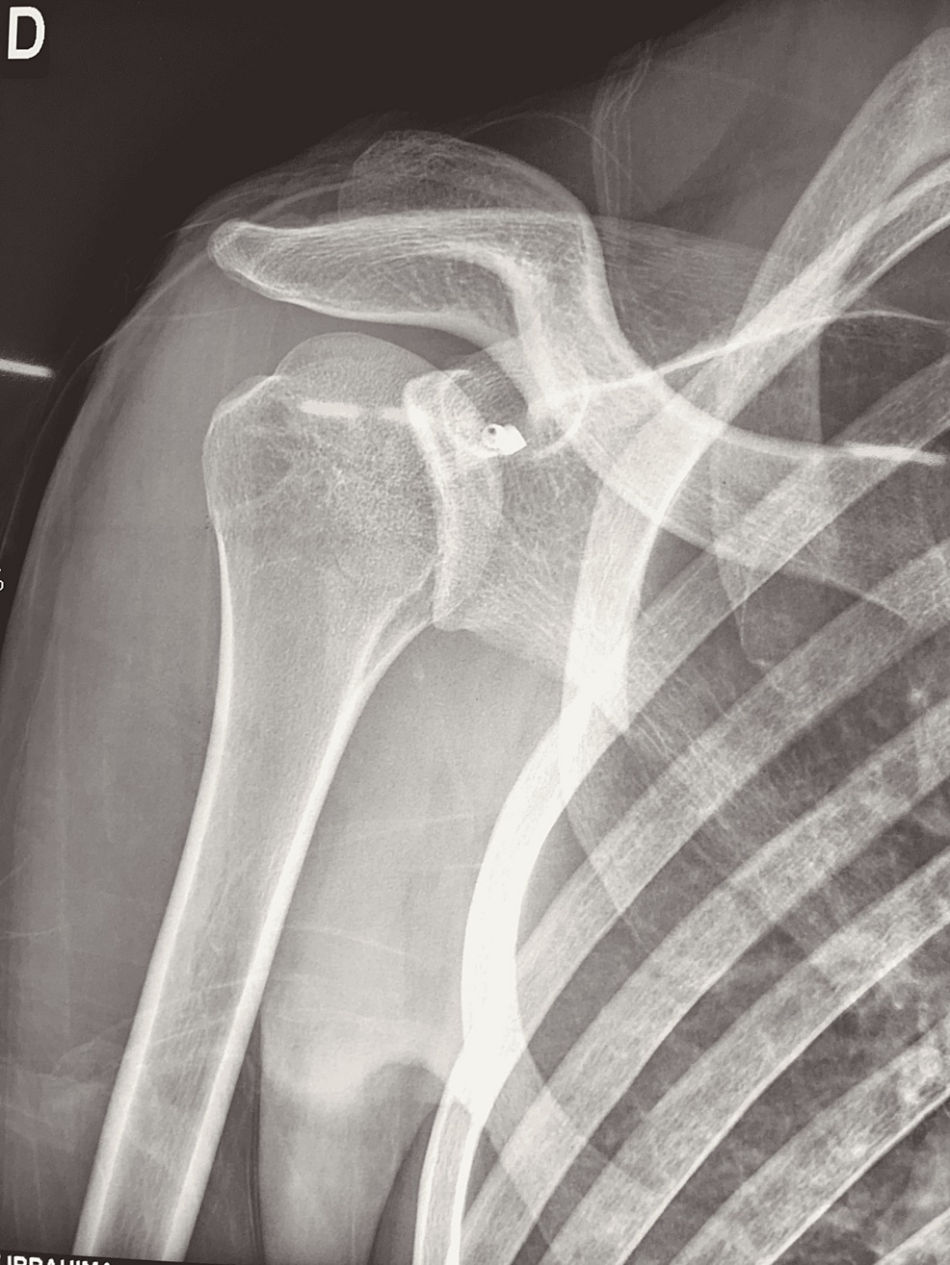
X-rays showing posterior dislocation of the right shoulder 15 days after the intervention.
